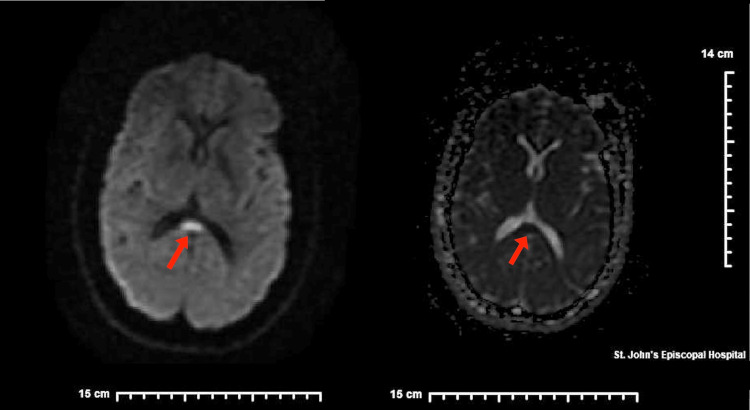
MRI T2-weighted axial DWI images from seizure admission containing lesions in the corpus callosum marked with red arrows. DWI: Diffusion-weighted imaging
